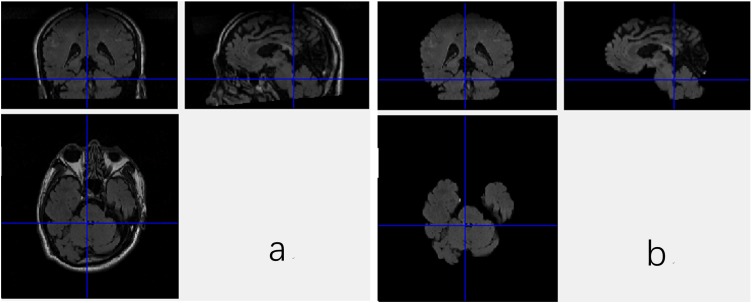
Three views of the MRI data with the non-brain tissues (a) and the data without non-brain tissues (b).
